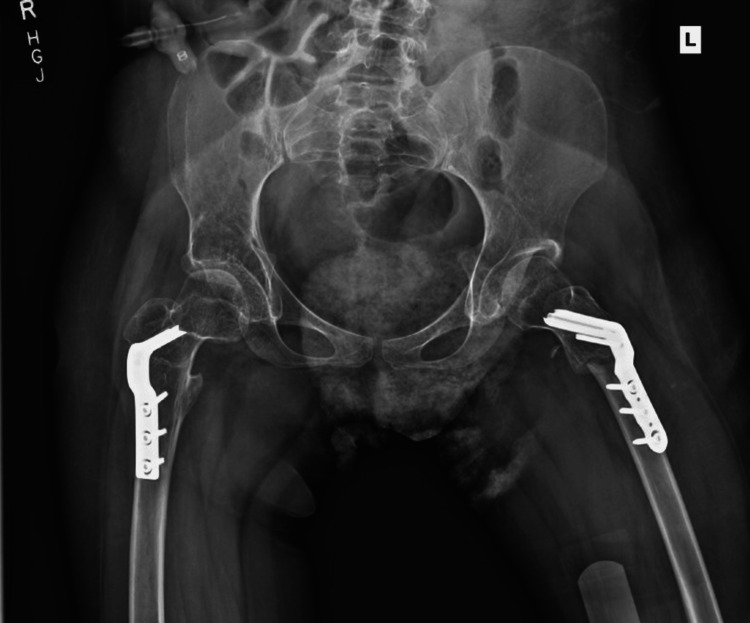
Postoperative radiographs of case 5, demonstrating the final alignment after bilateral hip adductor release and femoral varus derotation osteotomy
